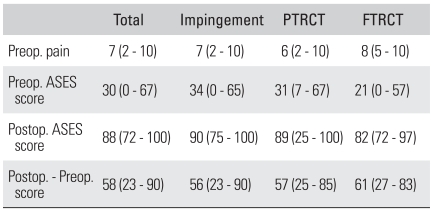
Clinical Data in Quantitative SPECT Study for Impingement Syndrome
